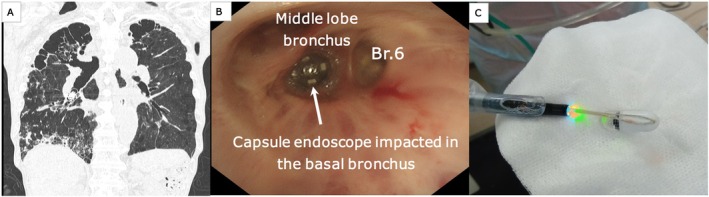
Impacted capsule endoscope. Plain chest CT (coronal view) reveals a foreign body in the right basal bronchus (A). A retained capsule endoscope is observed in the right basal bronchus (B). Retrieved/removed retained capsule endoscope (C).
